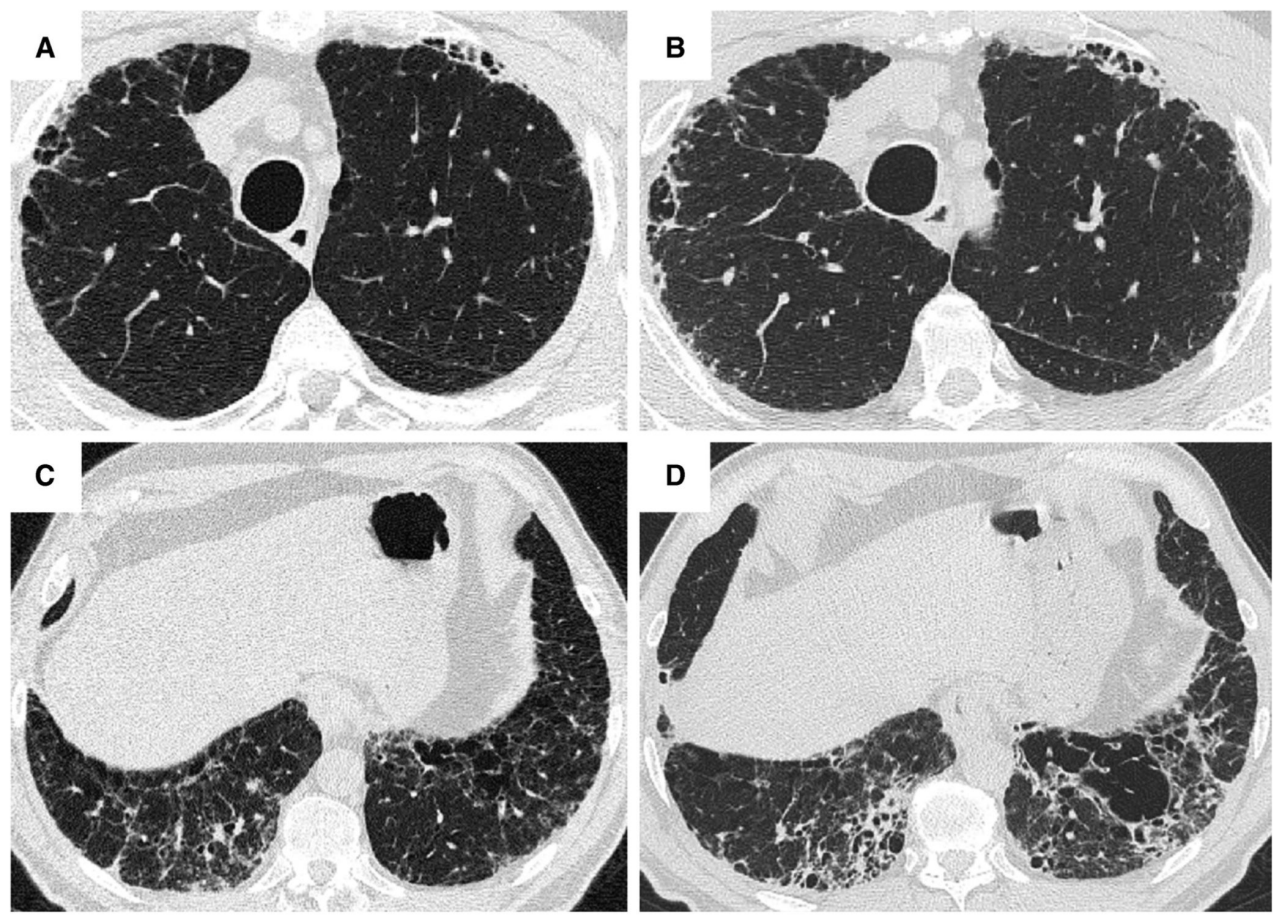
Chest HRCT showing worsening of admixed destructive emphysema (combined pulmonary fibrosis and emphysema, admixed pattern). In the pair of axial images of the upper (A, B) and lower zones (C, D), taken 2 years apart (A, C: baseline; B, D: follow-up) in a 66-year old male patient with idiopathic pulmonary fibrosis, isolated emphysema in the right upper lobe becomes admixed with fibrosis over time. In the left lower lobe, centrally placed emphysema becomes pulled apart (‘traction emphysema’) and expands as the surrounding fibrosis evolves.
